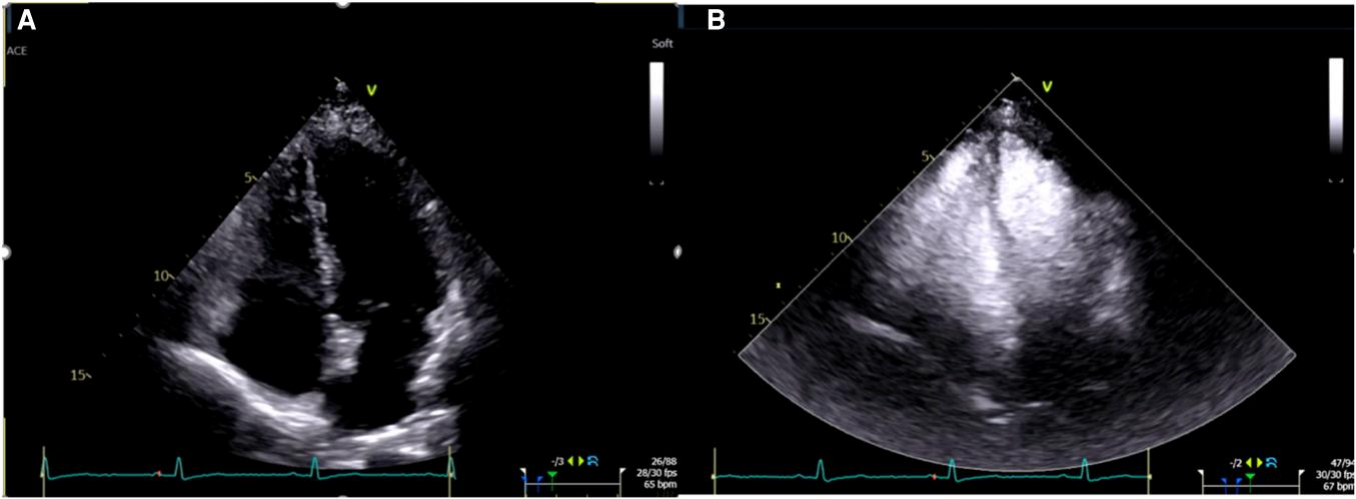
Non-contrast apical four-chamber view (*A*) and contrast-enhanced apical four-chamber view (*B*) on transthoracic echocardiogram.
